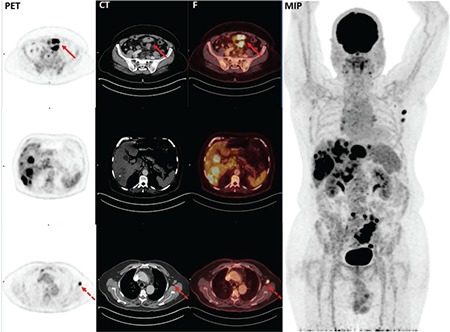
A 65-year-old male patient with mutant type adenocarcinoma localized to sigmoid colon positron emission tomography, computed tomography, fusion, maximum intensity projection. Red arrows show fluorodeoxyglucose uptake of the primary tumor, primary tumor: SUV_max_: 31.5 with multiple liver metastases. Dotted red arrows show left axillary lymph node metastases. SUV_max_: Maximum standardized uptake value, PET: Positron emission tomography, CT: Computed tomography, F: Fusion, MIP: Maximum intensity projection
